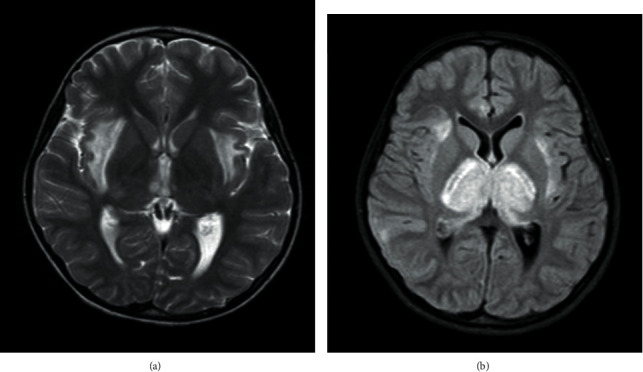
MRI images of case 1 at the (a) first and the (b) second admission.
